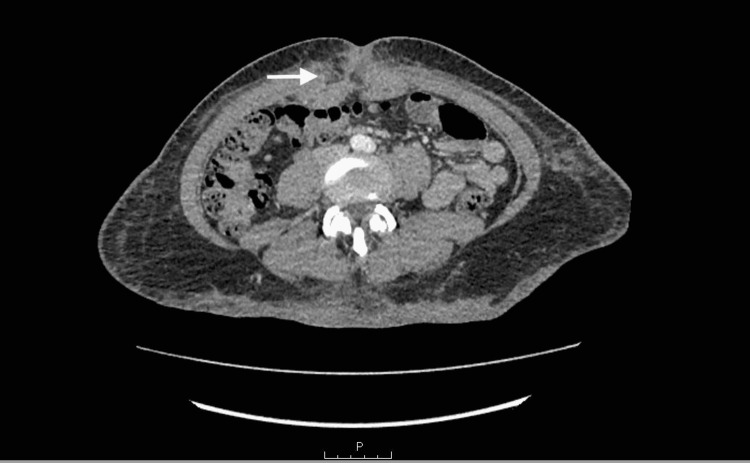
CT abdomen and pelvis with IV contrast The arrow shows diffuse abdominal wall subcutaneous stranding with no abdominal wall abscess.
